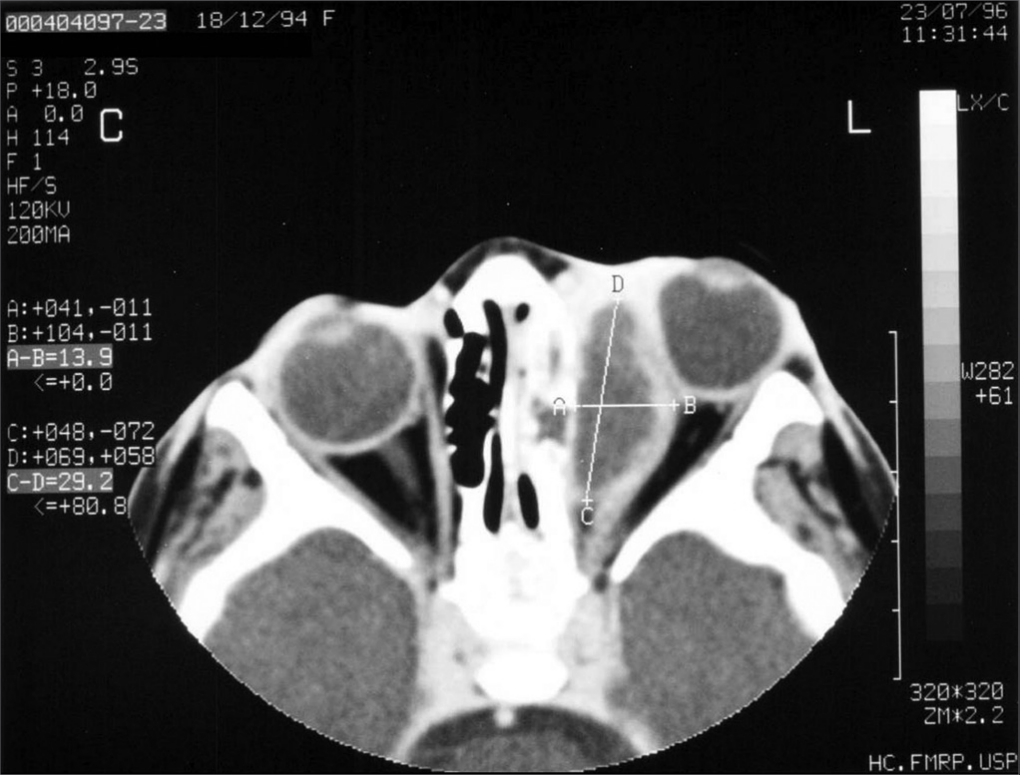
Axial view CT scan of the orbit showing subperiosteal abscess on the left side.
